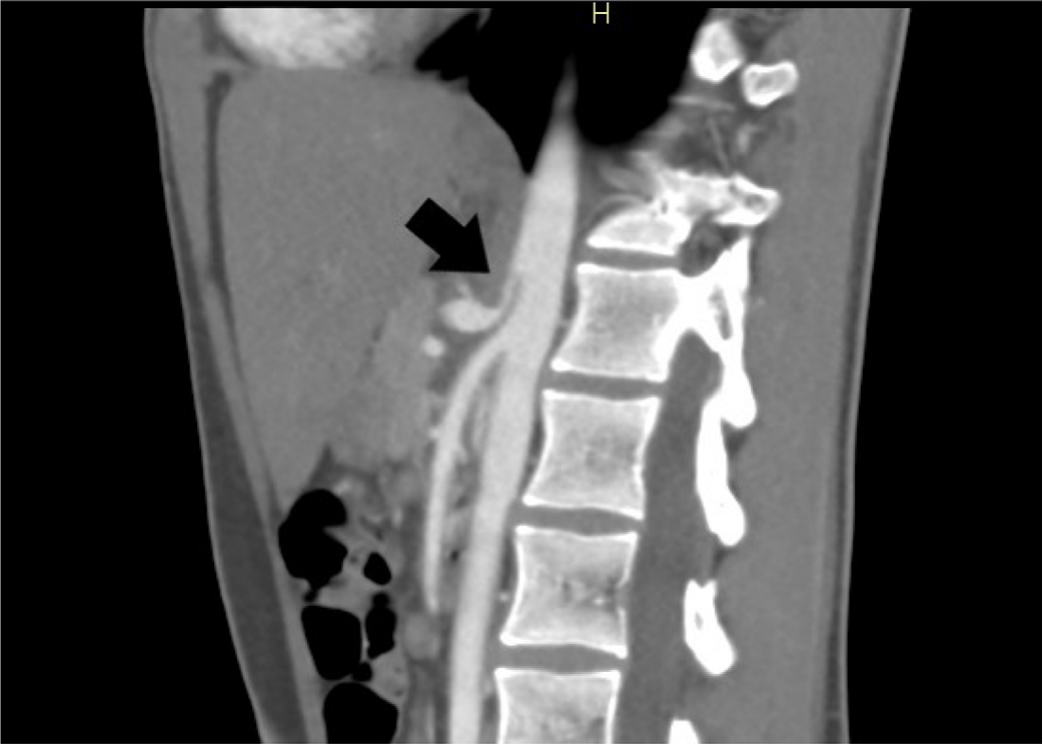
Computed tomography angiography abdomen and pelvis demonstrating narrowing of the celiac artery in a J-shaped configuration with post stenotic dilation seen in median arcuate ligament syndrome (arrow).
